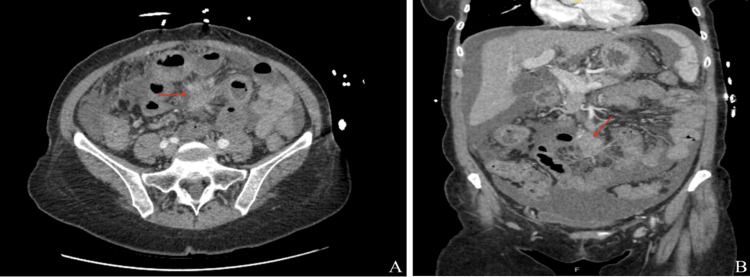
Axial (A) and sagittal (B) views of CT abdomen/pelvis demonstrating an ill-defined mesenteric soft tissue mass
